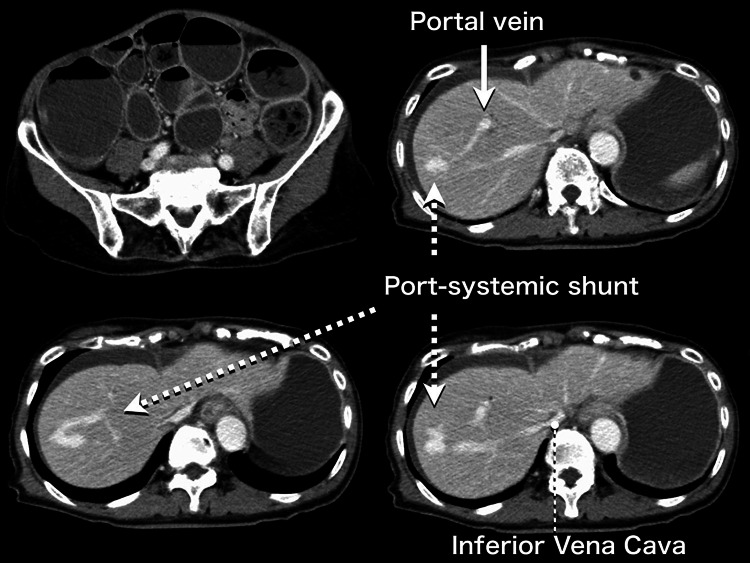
Fecal bowel obstruction and congenital portosystemic shunt Computed tomography with contrast showed a portosystemic shunt (dotted arrow) between the right hepatic vein and portal vein (solid arrow), as well as bowel obstruction by feces filled up from the rectum to the descending colon, with gastrointestinal tract distention up to the stomach without any luminal filling deficit.
